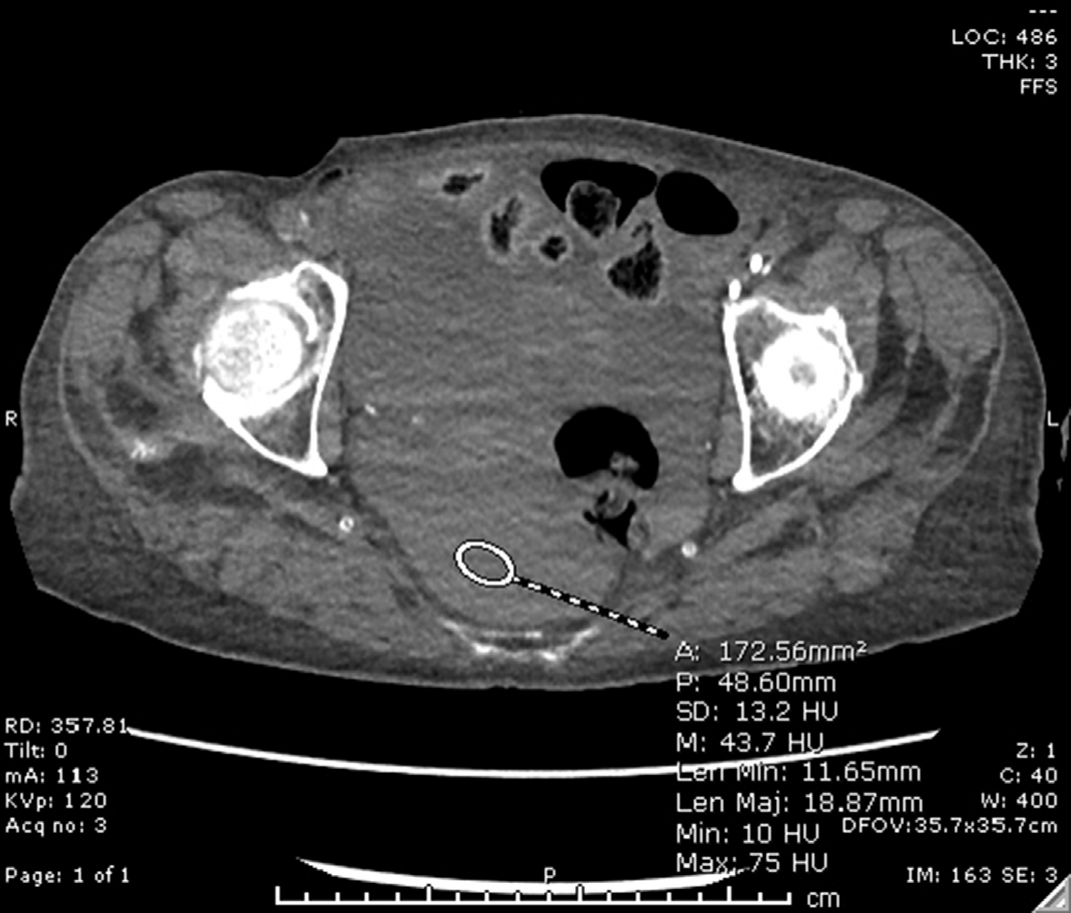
Noncontrast Computed Tomography of Abdomen View showing hemoperitoneum.
